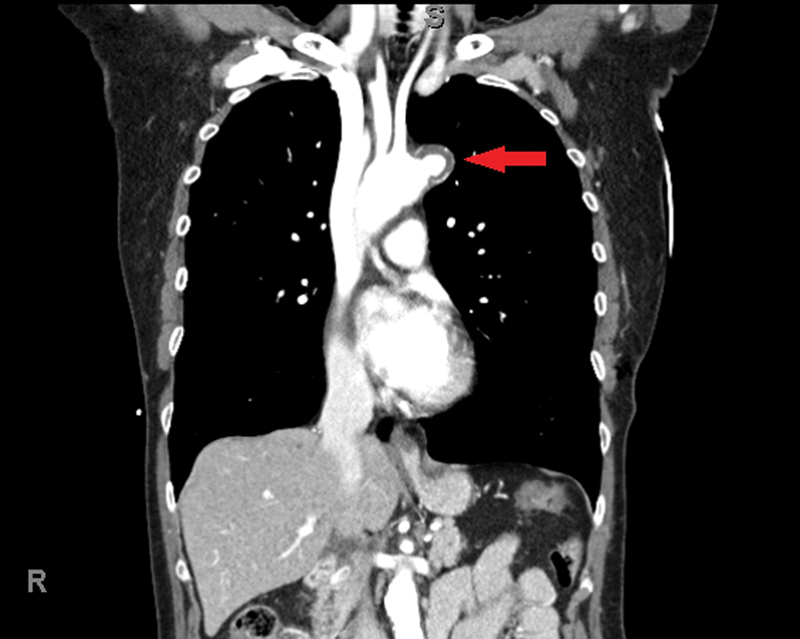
Computed tomography (CT) aorta showing the pseudoaneurysm.
